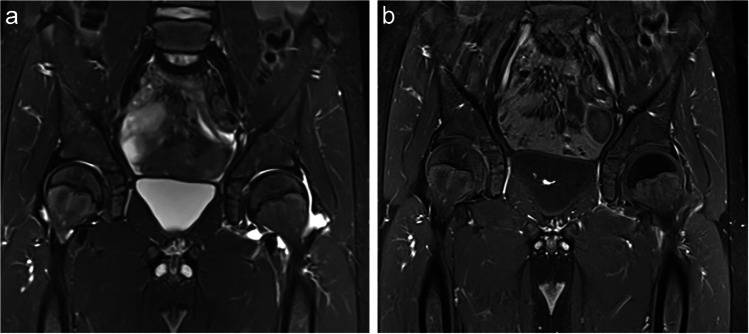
A 6-year-old boy with transient synovitis. The patient developed flu-like symptoms 10 days before admission and presented with 1 day of left hip pain, unable to bear weight on the day of admission. He was afebrile, with a white blood cell (WBC) count of 12,400 cells/mL, an erythrocyte sedimentation rate (ESR) of 43 mm/h, and a C-reactive protein (CRP) level of 3.5 mg/dL. Left hip aspiration yielded a WBC count of 1,150 cells/mL with a negative culture. He was treated with a non-steroidal anti-inflammatory drug (NSAID), resulting in symptom improvement. A coronal MRI STIR shows a large left hip effusion. B coronal MRI SET1 with fat suppression post-contrast shows decreased enhancement of the left femoral head
